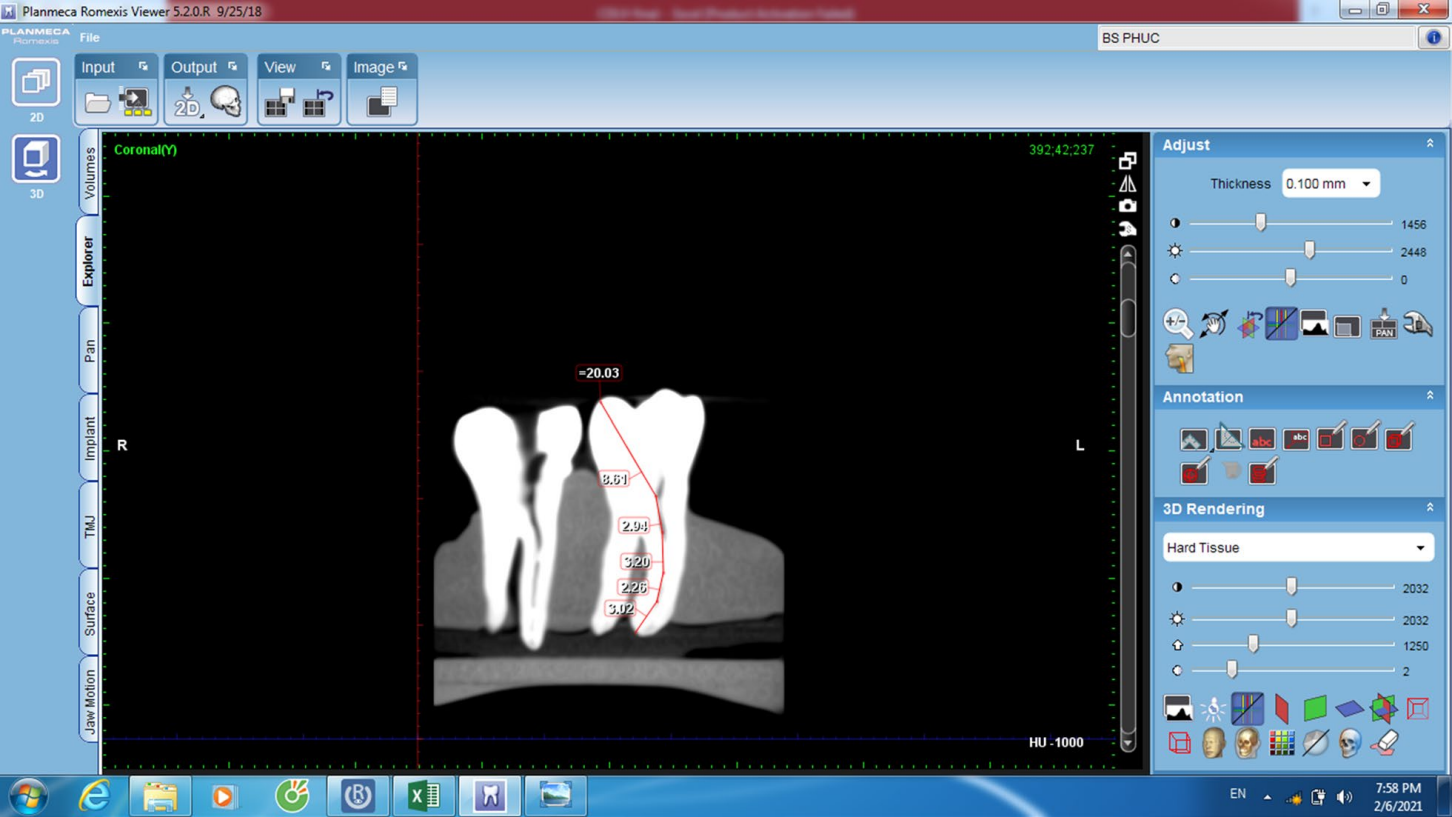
Romexis Viewer measurement on the CBCT software
